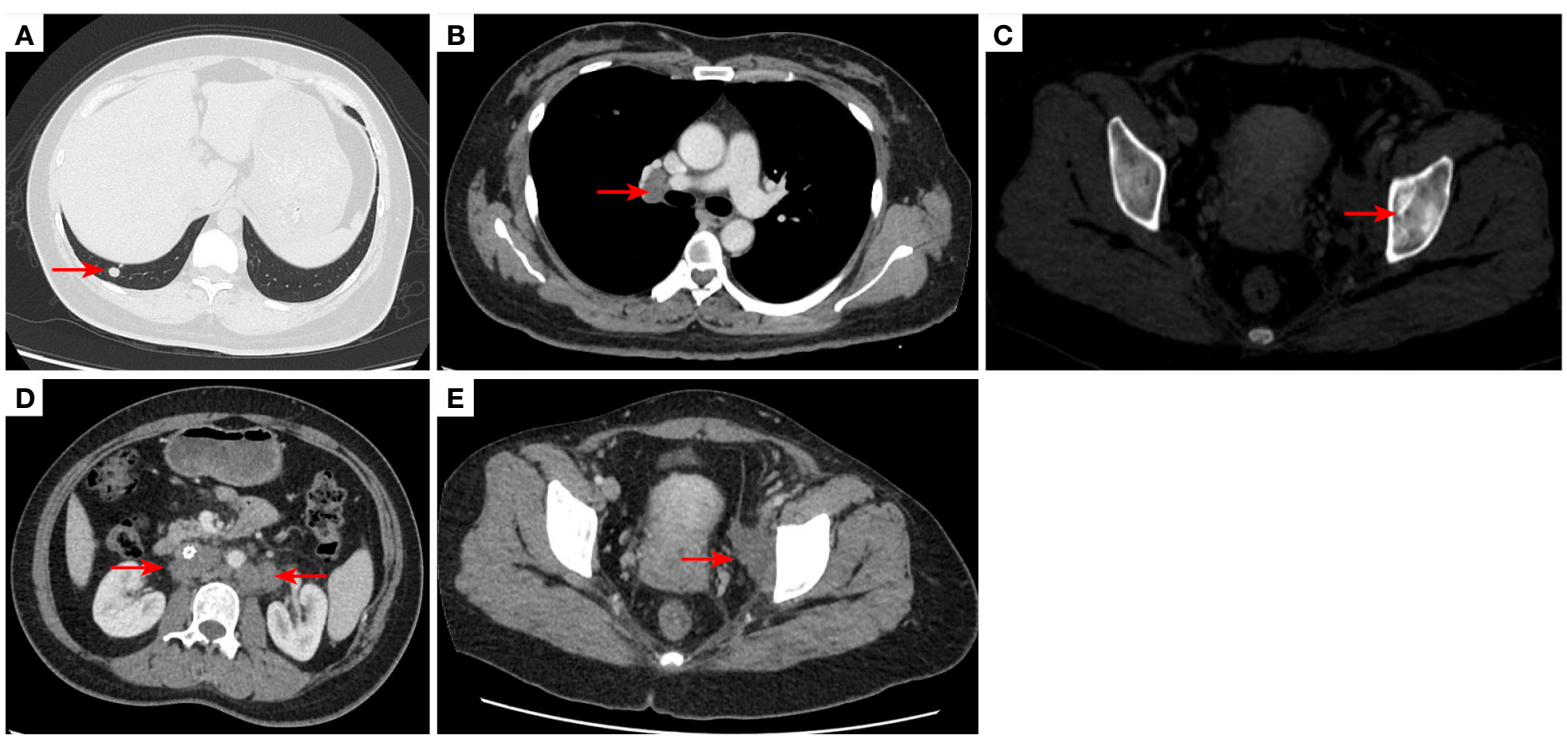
2020–12-22 CT scan: The right lower lobe of the lung metastatic tumor reappeared [red arrow, (A)] and the long diameter was 0.91 cm short diameter of right hilar lymph node was 1.66 cm [red arrow, (B)]; Left acetabular metastases [red arrow, (C)]. the short diameter of retroperitoneal lymph nodes was 1.66 cm, and the short diameter of left pelvic lymph nodes was 3.45 cm [red arrow, (D, E)]. The efficacy was PD. PD, progressive disease.
